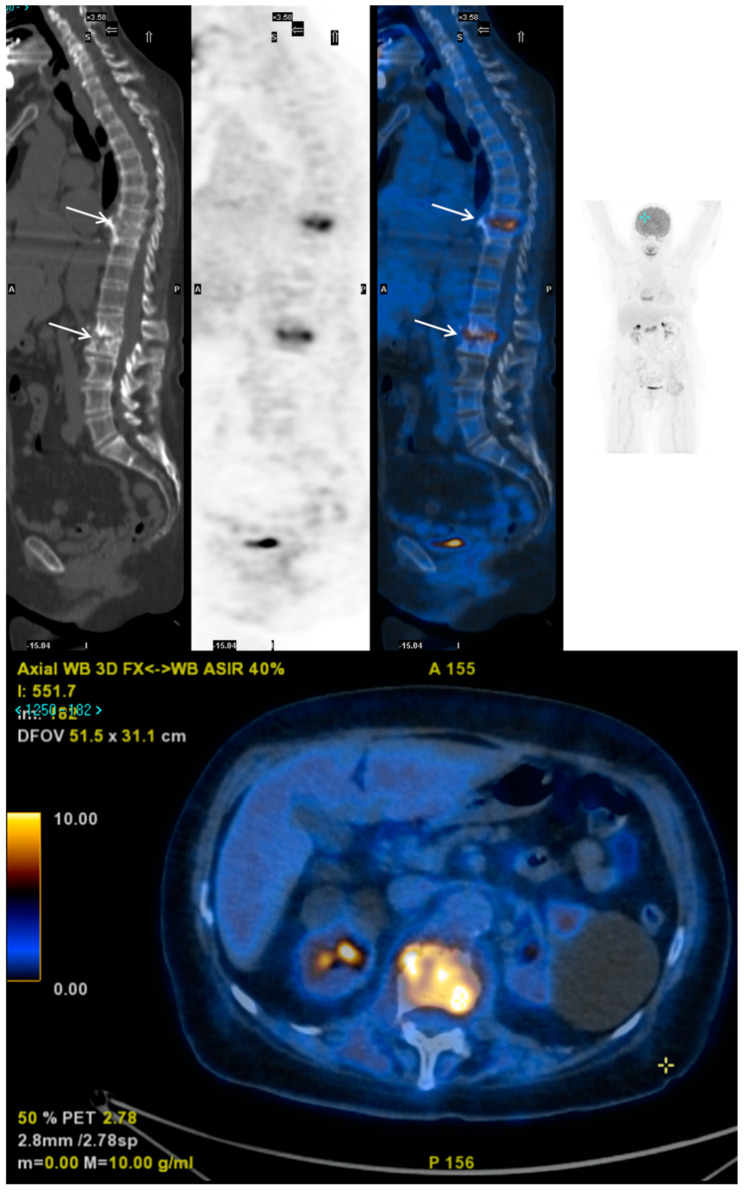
Pyogenic bifocal spondylodiscitis (T8-T9 and L1-L2) in a 70-year-old woman. FDG PET-CT showed increased SUV on both vertebral levels (arrows).
